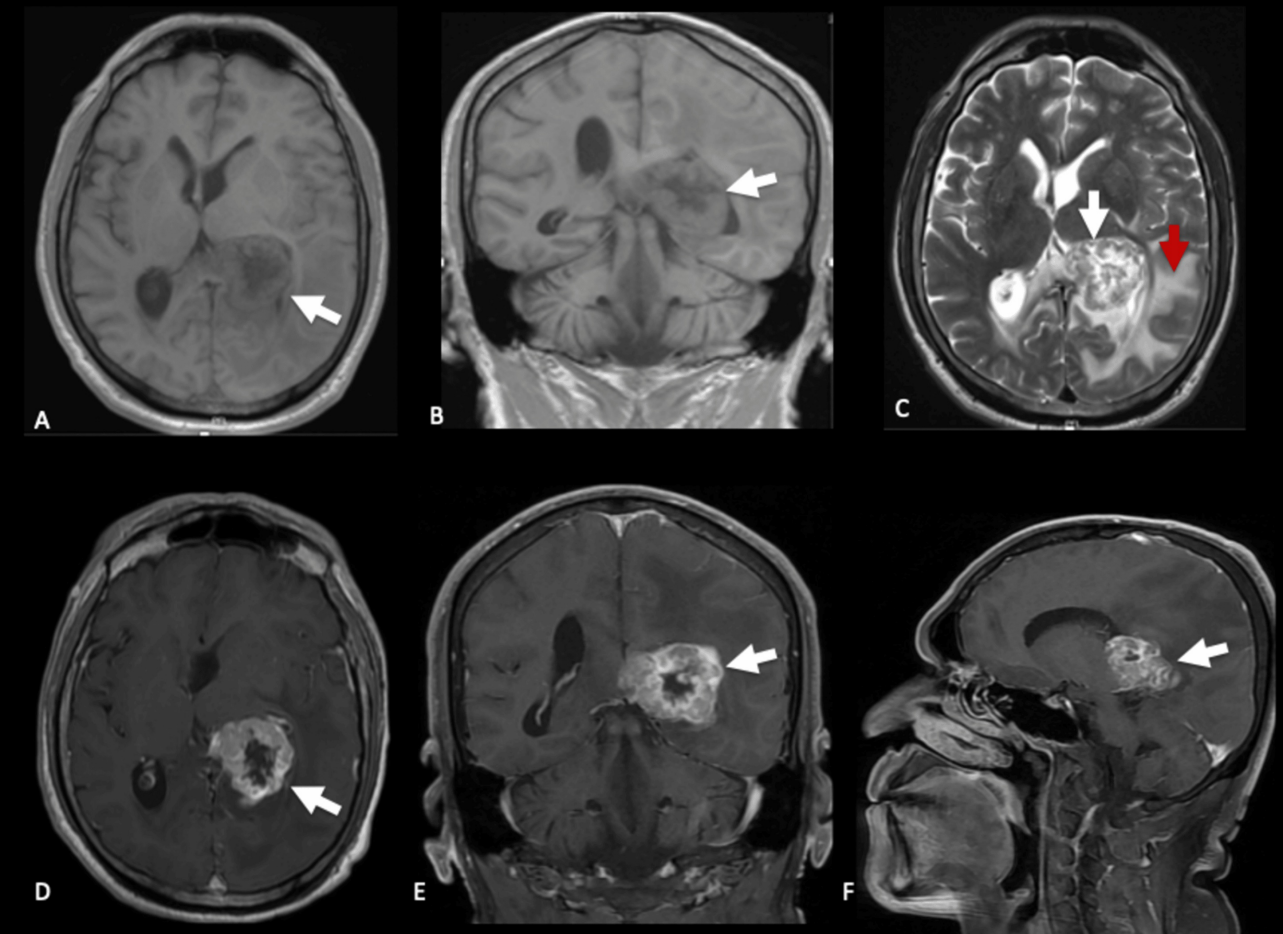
Preoperative brain MRI (A, B) Axial and coronal non-contrast T1-weighted images showing a hypointense, heterogeneous intraventricular mass in the left lateral ventricle atrium (white arrows). (C) Axial T2-weighted image demonstrating mixed signal intensity with hypointense and hyperintense components (white arrow) and surrounding vasogenic edema (red arrow). (D–F) Axial, coronal, and sagittal post-contrast T1-weighted images depicting heterogeneous peripheral enhancement (white arrows), consistent with necrotic or hemorrhagic tumor areas.
